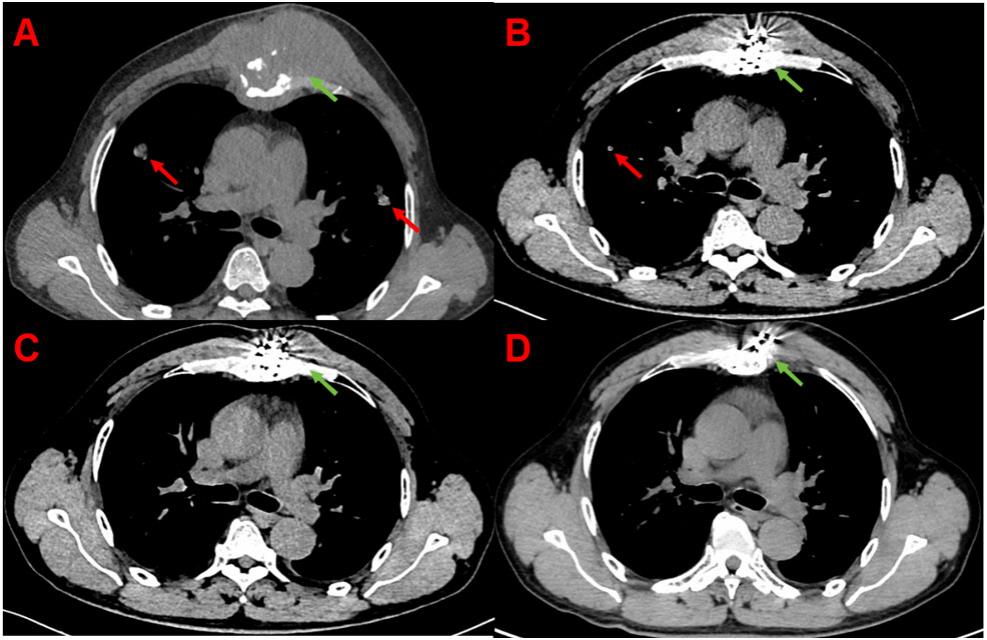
Serial CT images of a representative HCC patient with a thoracic vertebral bone metastasis, before and after combination therapy. (A) Baseline image shows a destructive metastasis in a thoracic vertebra (arrow). (B) 3 months after PD-1 inhibitor plus ^125^I seed implantation into the lesion, the metastatic tumor has regressed significantly. (C) 6 months post-treatment, only a small residual lesion remains. (D) 12 months post-treatment, the treated bone bone metastasis showed almost complete resolution with no evidence of recurrence. The patient experienced substantial pain relief and no neurological complications following treatment.
